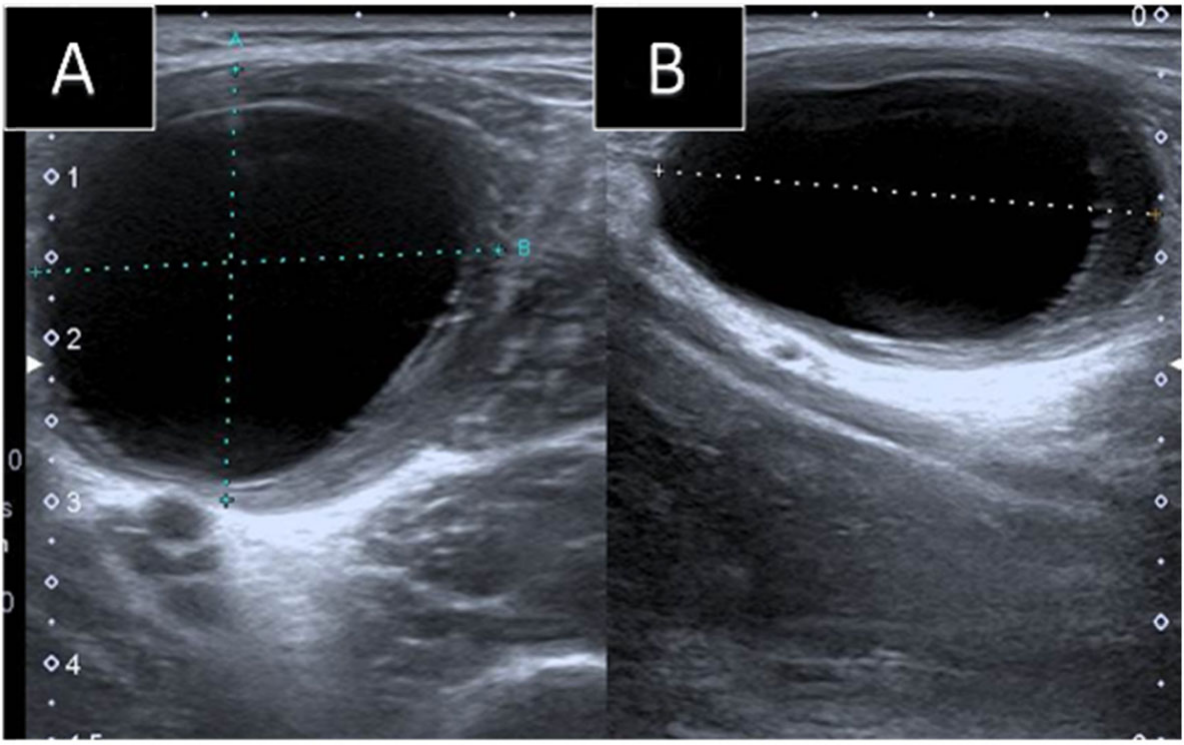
Ultrasound images of the left thigh. a: Trans, b: longitudinal: Showing a well-defined homogenous cystic lesion in the left rectus femoris muscle with posterior acoustic enhancement seen
